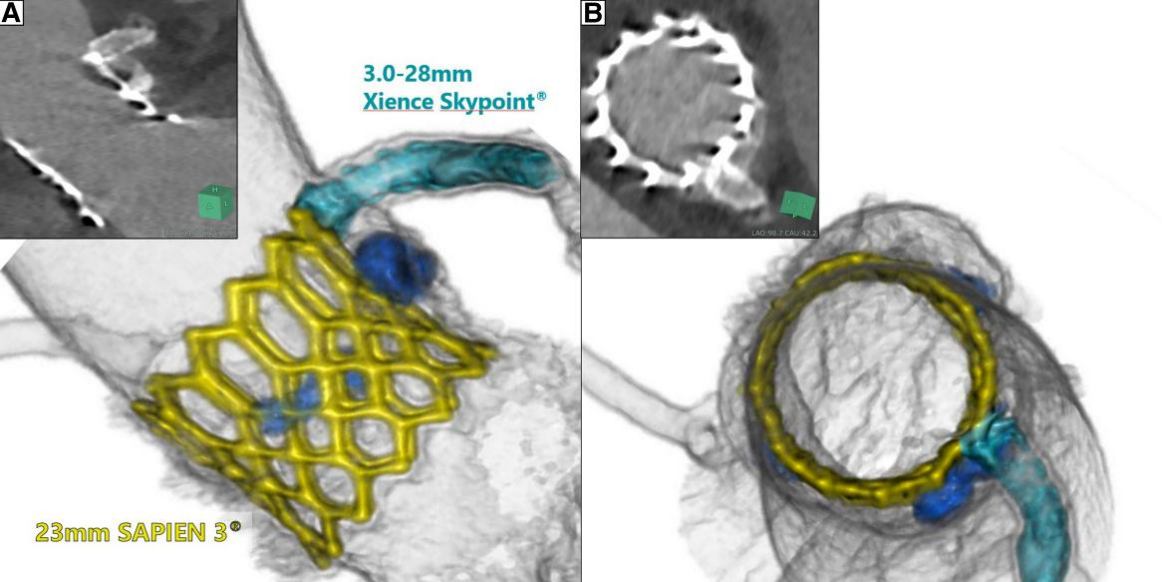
The transcatheter heart valve and the stent in the left main coronary artery on computed tomography 3 days after transcatheter aortic valve replacement. THV, transcatheter heart valve; LMCA, left main coronary artery; CT, computed tomography; TAVR, transcatheter aortic valve replacement.
